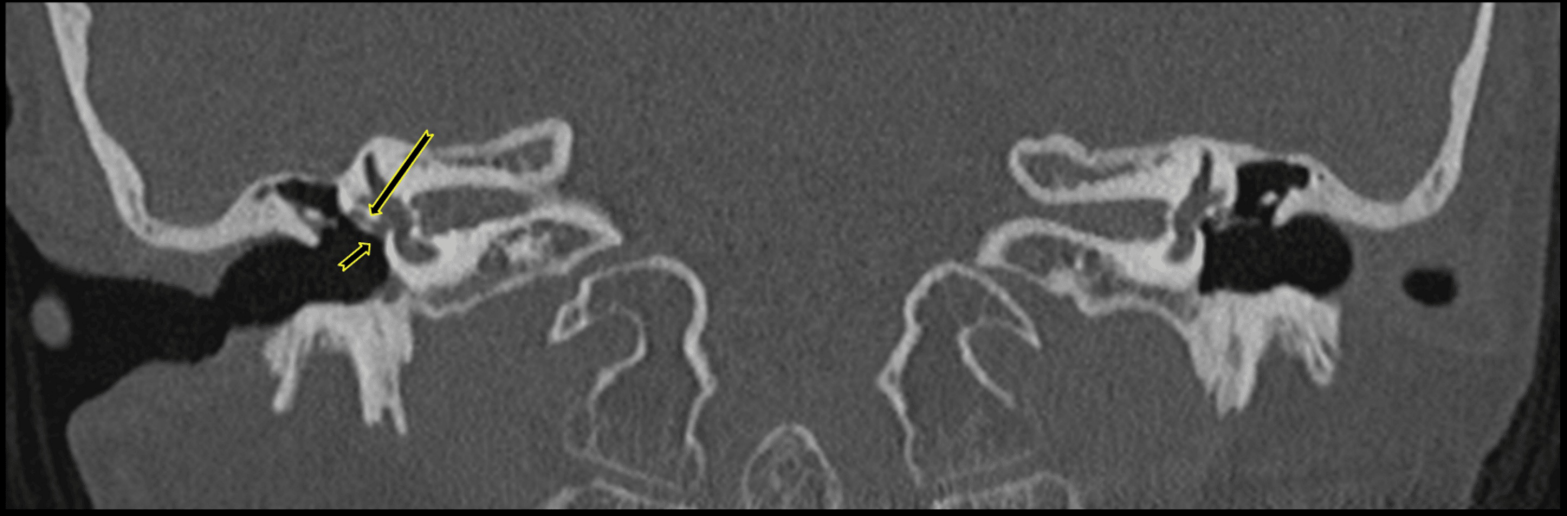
Temporal bone coronal CT reconstructions demonstrate the small vascular channel of the PSA obliterating and crossing the right oval window. The PSA obliterates and crosses the right oval window (short arrow). It passes through a small channel (long arrow) toward the lower infero-medial aspect of the tympanic segment of the facial nerve canal. PSA: persistent stapedial artery
